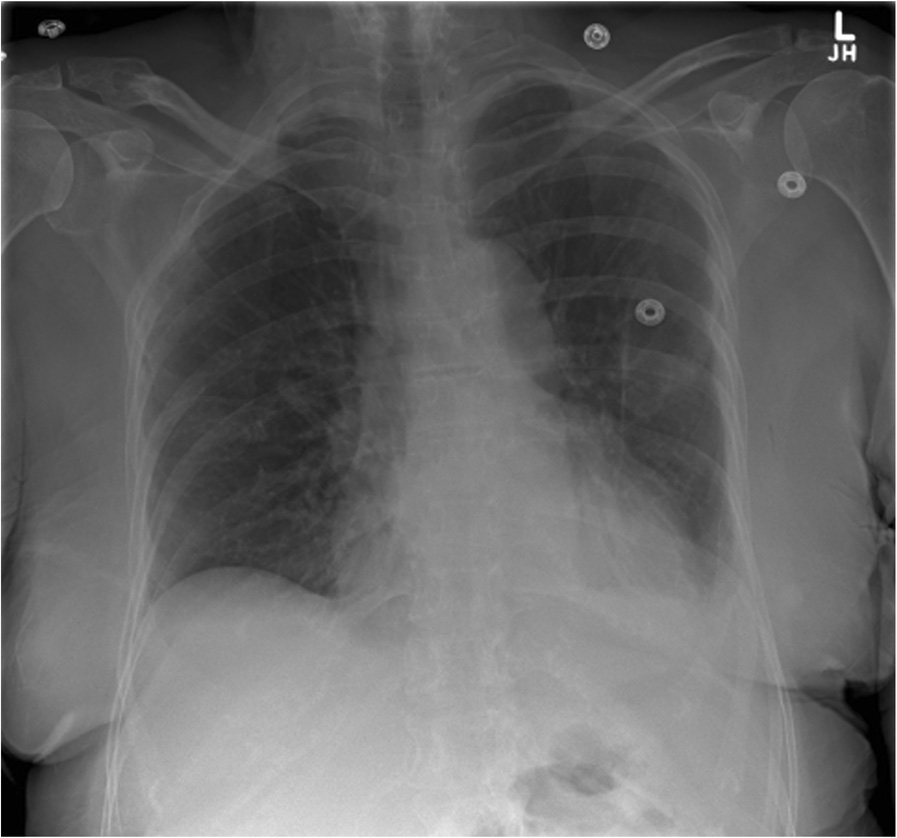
Case 7: chest radiograph obtained after removal of a left-sided tube thoracostomy. The chest radiograph noted a ‘small focal lucency at the right apex that could represent a small loculated pneumothorax.’
